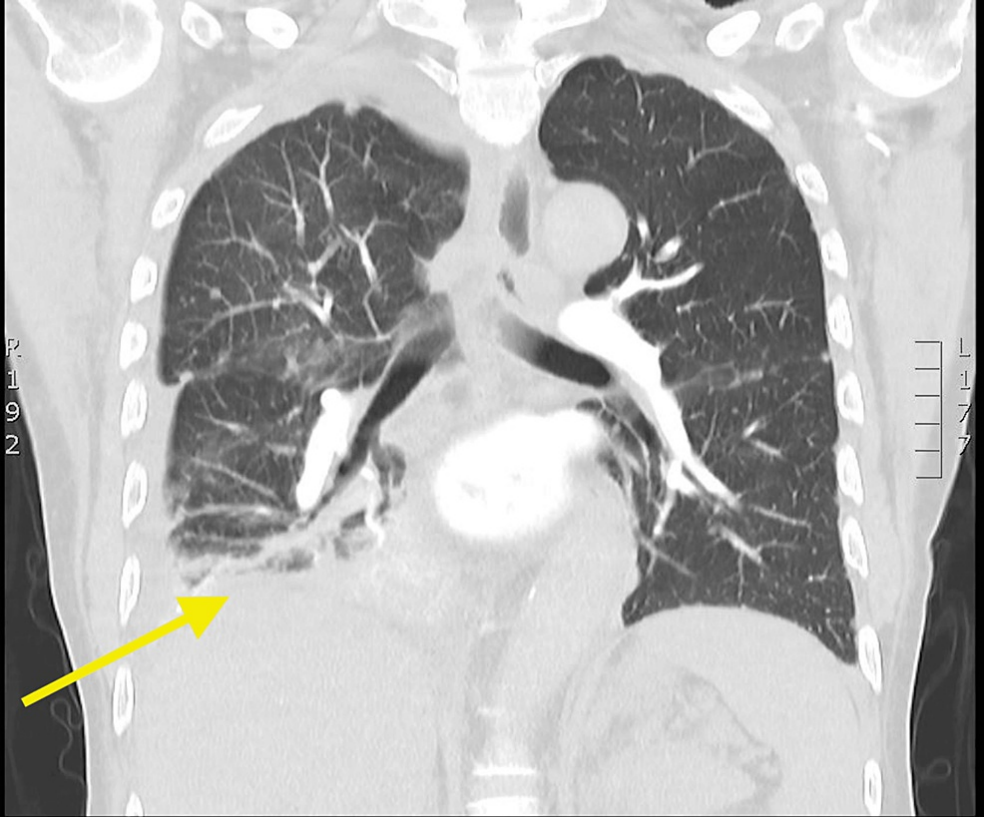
Coronal CT images of right-sided pleural effusion with small military metastases in the lung CT = computed tomography
